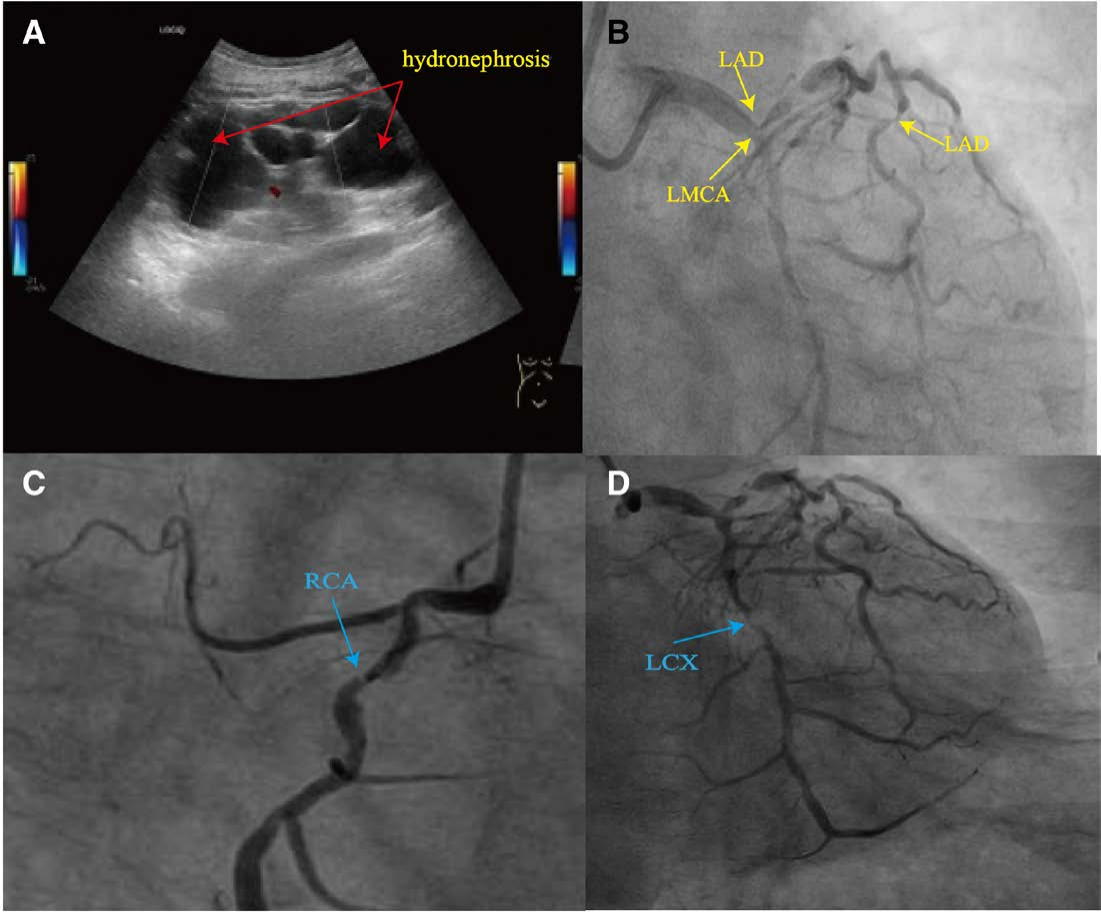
(A) Urological ultrasound at presentation: the renal pelvis and calyces of the left kidney were dilated with thin parenchyma, the collecting system was dilated with a width of about 34 mm, and several strong echoes were seen in the calyces, the larger of which was about 13 × 4 mm in size, accompanied by acoustic shadows. A strong echo of about 17 × 7 mm with acoustic shadow was detected in the upper part of the left ureter, and the ureter above it was dilated. (B) The first CAG examinations: there was 60% stenosis of the LMCA, 90%–95% stenosis of the LAD artery. (C) 90% proximal stenosis of the right coronary artery. (D) 95% stenosis of the LCX. CAG = coronary angiography, LAD = left anterior descending, LCX = left circumflex artery, LMCA = left main coronary artery.
